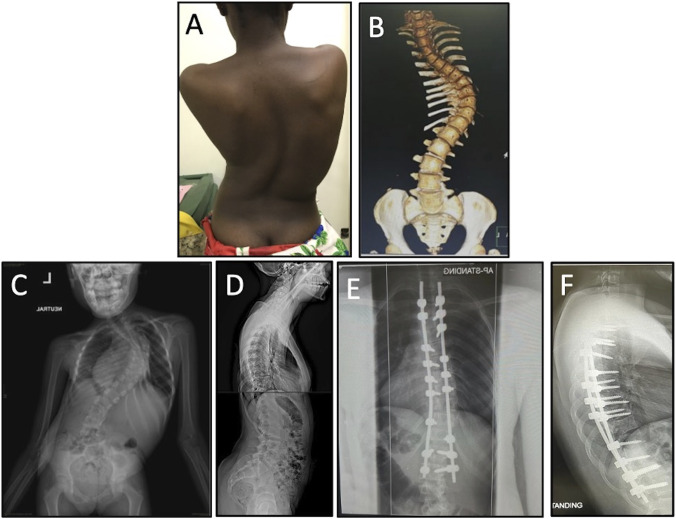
Example of a patient operated on by visiting surgeons and participants of the on-site camp at Muhimbili Orthopaedic Institute. A, Photograph of a patient in clinic with adolescent idiopathic scoliosis. Note the dextroscoliotic curve of the spine, unlevel shoulders, prominent shoulder blade, rib hump, and mild pelvic obliquity. B, Preoperative 3-dimensional reconstruction. C, PA radiograph demonstrating almost a 90-degree main thoracic curve with axial rotation. D, Preoperative lateral radiograph without any significant malalignment. Postoperative (E) PA and (F) lateral radiographs status post T3-L2 instrumented fusion with posterior column osteotomies, demonstrating deformity correction with straightening of the coronal curve and axial derotation. There were no intraoperative or postoperative complications.
